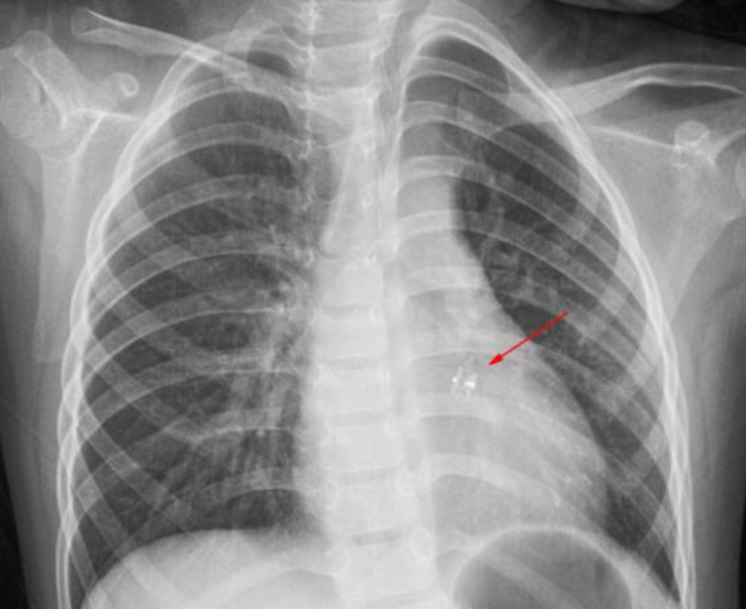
Chest X-ray showing the position of the occluder after occlusion of the sinus of Valsalva-right ventricle fistula (arrow)
